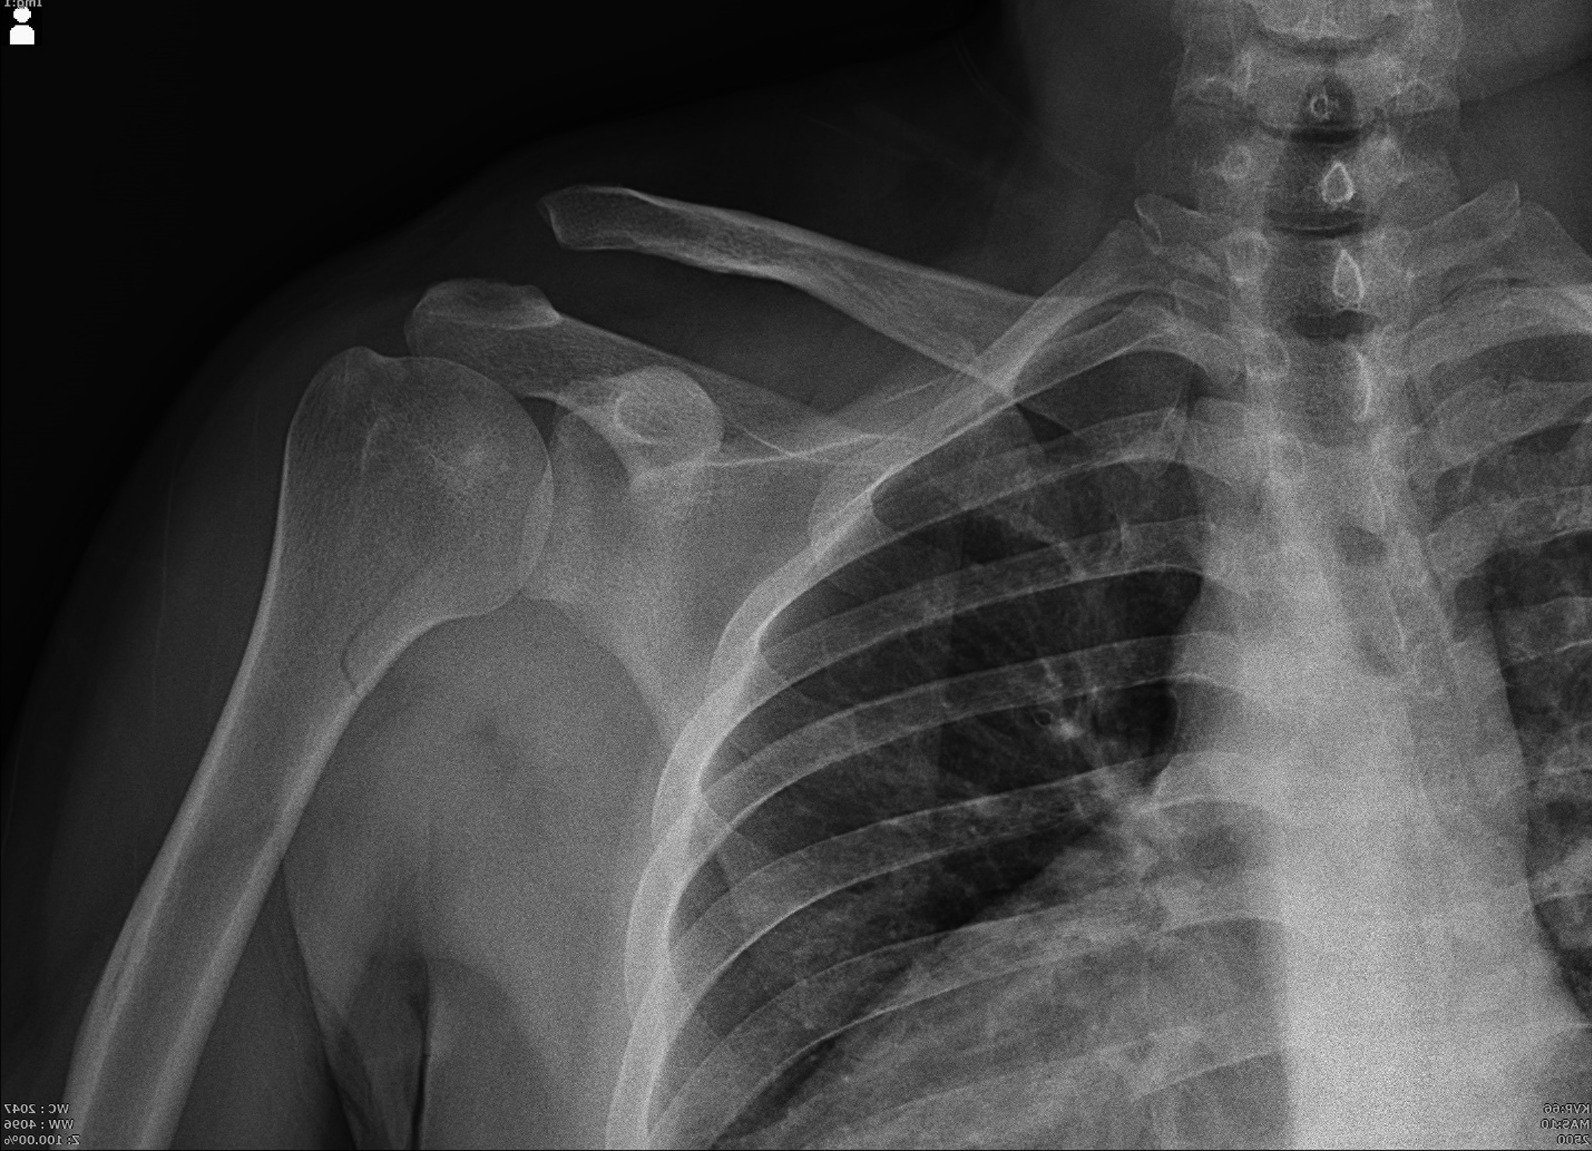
Concomitant Rockwood type V ACJ dislocation and a non-displaced unicortical proximal humerus fracture in digital X-ray
